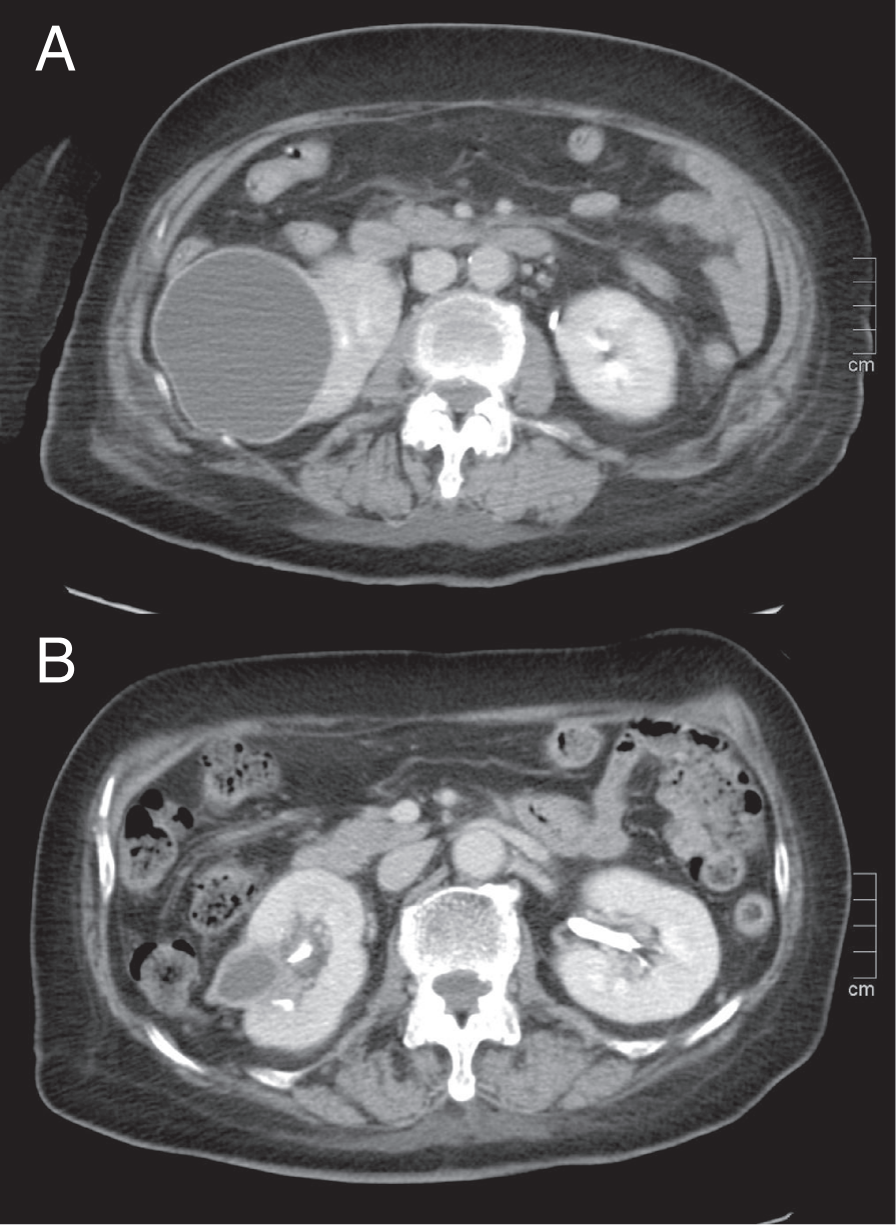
Contrast-enhanced computed tomography (CT) images of the abdomen on days 6 and 35. A: CT image on day 6 showed a right renal cyst (9.3 × 7.2 cm). The CT attenuation value of the inner cyst contents was about 25 Hounsfield units, much higher than the value of water. The lateral margin of the cyst was faintly contrasted. B: CT image on day 35. The right renal cyst had markedly decreased in size after the drainage procedure.
